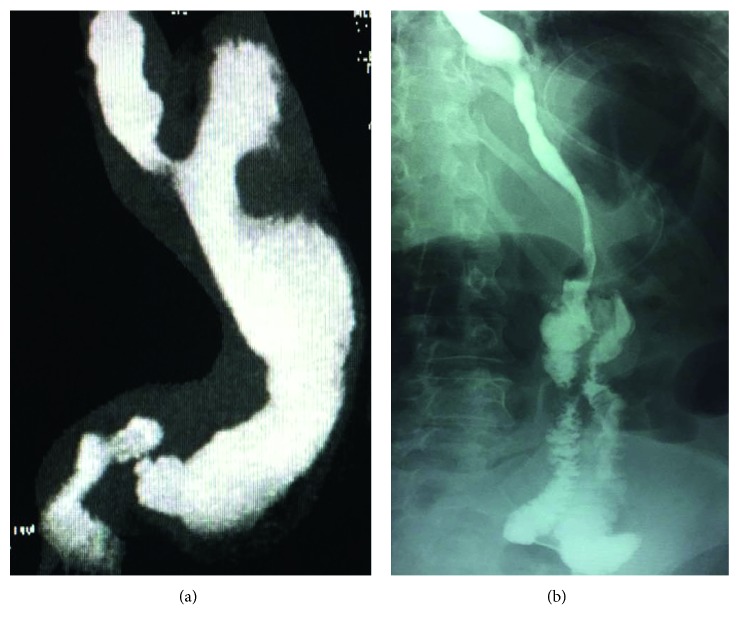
(a) Gastric prolapse following gastric plication; (b) postoperative X-ray swallow examination showing sleeve gastrectomy with omega loop anastomosis.
